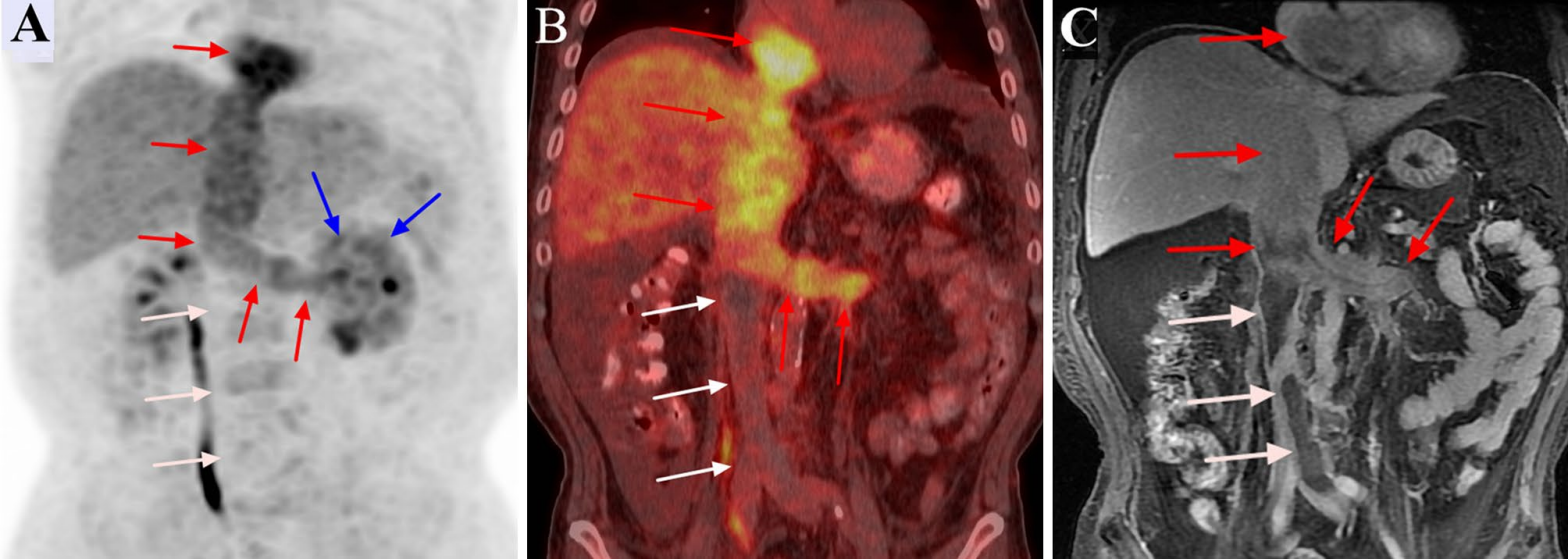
^18^F-FDG positron emission tomography (PET)/computed tomography (CT) and contrast-enhanced MRI (CEMRI) scans of a 67-year-old man who had clear cell carcinoma of the left kidney with a level IV venous tumour thrombus (VTT) and venous bland thrombus (VBT). The VTT had invaded the venous wall and was graded as level IV by both PET/CT and CEMRI. The site of diffusely increased FDG uptake (SUVmax 5.3) on the PET maximum intensity projection image is the primary tumour (blue arrow) (a). The site of pathologically increased FDG uptake (SUVmax 5.7) on the PET and PET/CT images from the left renal vein to the inferior vena cava is the VTT (red arrow) (a, b). The focal site of decreased FDG uptake (SUVmax 1.2) at the inferior vena cava is the VBT (white arrow) (a, b). The VTT with a regular shape shows heterogeneous enhancement and completely occludes the inferior vena cava lumen on coronal CEMRI (red arrow) (c), while the VBT shows no enhancement (white arrow) (c).
